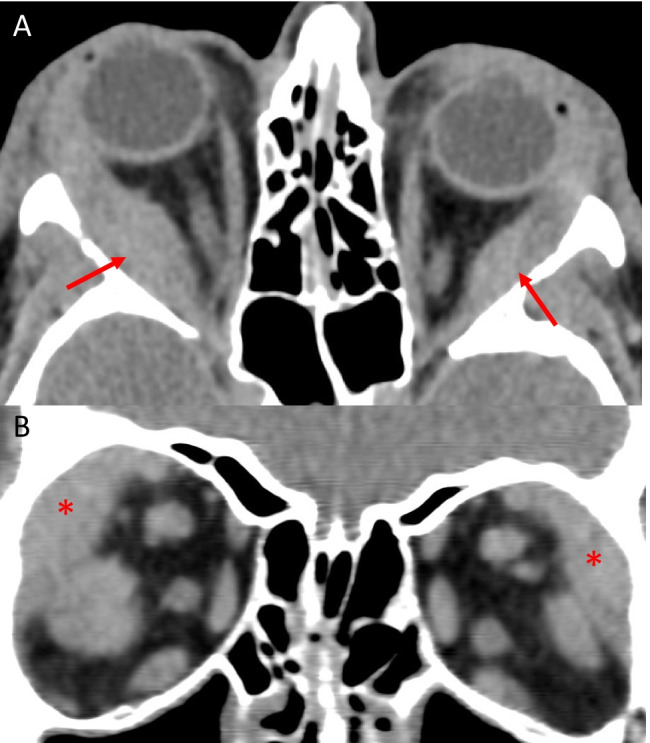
IgG4-related ophthalmic disease. Axial CT (A) showing bilateral enlargement of the lateral recti (R > L) including the anterior tendon (arrows). Coronal CT (B) demonstrating bilateral enlargement of the lacrimal glands (*)
